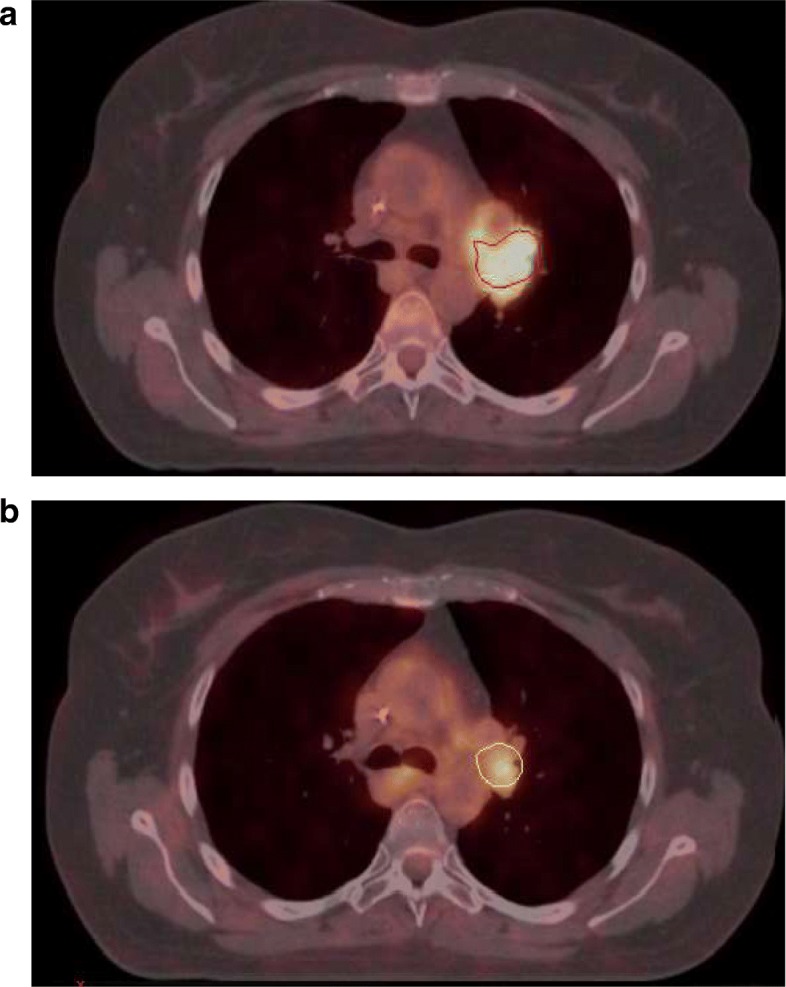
Patient with stage IIIA left lung adenocarcinoma. FDG PET/CT performed before (a.) and during (b) radiation therapy. Pre therapeutic scan (a) show left para-hilar hypermetabolism with SUV_max_ = 9.6 and MTV = 15.4 cc defined with a threshold value of 41% SUV_max_. Per-therapeutic data (b) reveals a decrease in FDG uptake (SUV_max_ = 4.2) and MTV = 4.8 cc. defined by the experts with a threshold value of 55% of SUV_max_
